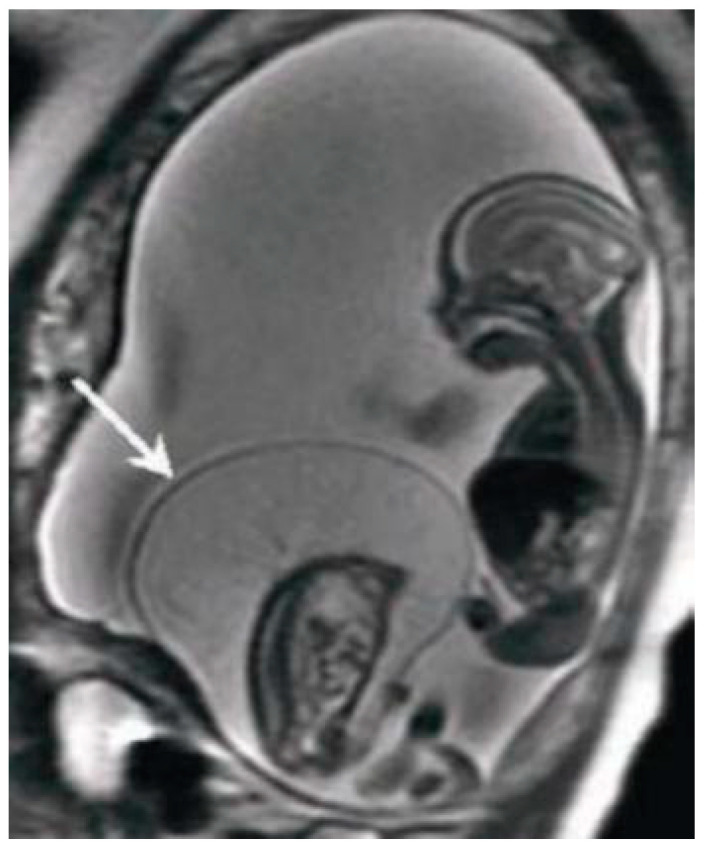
Fetal magnetic resonance imaging (MRI) of Twin reversed arterial perfusion (TRAP) sequence. Half-Fourier Acquisition Single-Shot Turbo-Spin-Echo (HASTE) T2 Sag. Both the acardiac (signaled by the arrow) and pump twins are visible.
